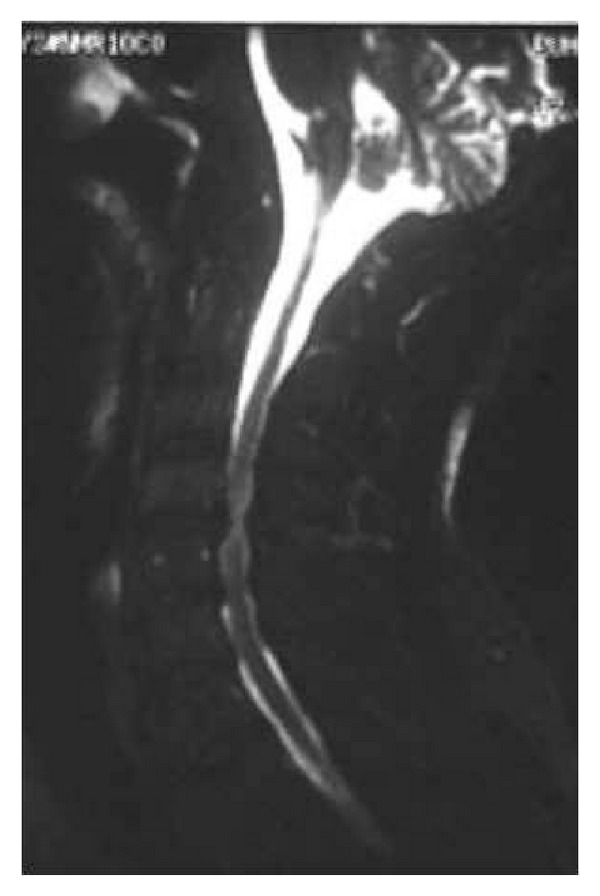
Lateral T2-weighted MRI of the cervical spine denoting significant spondylotic changes.
